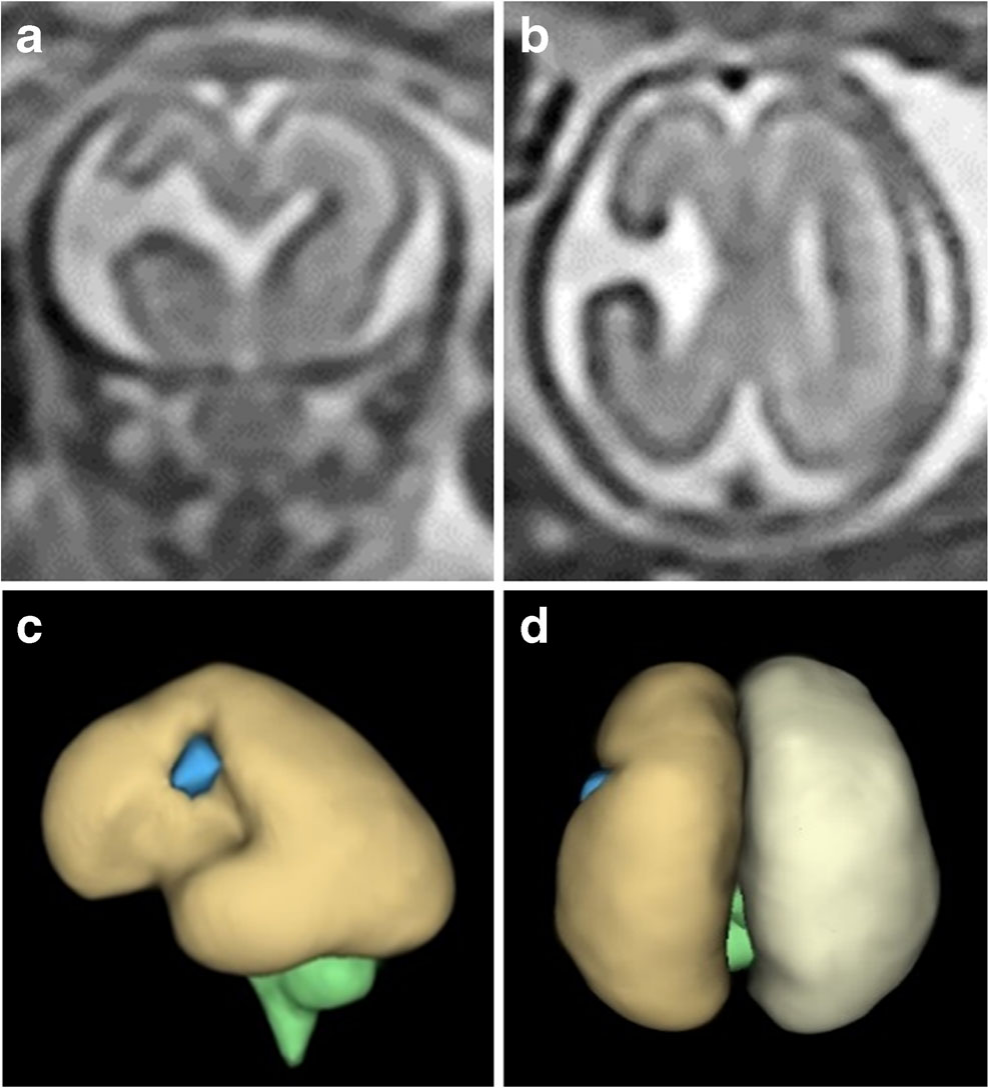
Unilateral schizencephaly (type 3) in a 21gw fetus. Coronal (a) and axial (b) ultrafast T2-weighted images show a widely spaced cleft in the right paracentral lobule. Those images are reversed to be consistent with the constructed models (c—left lateral and d—superior) of the brain constructed from a 3D steady-state acquisition. The septum pellucidum is absent but no other brain abnormality was shown
